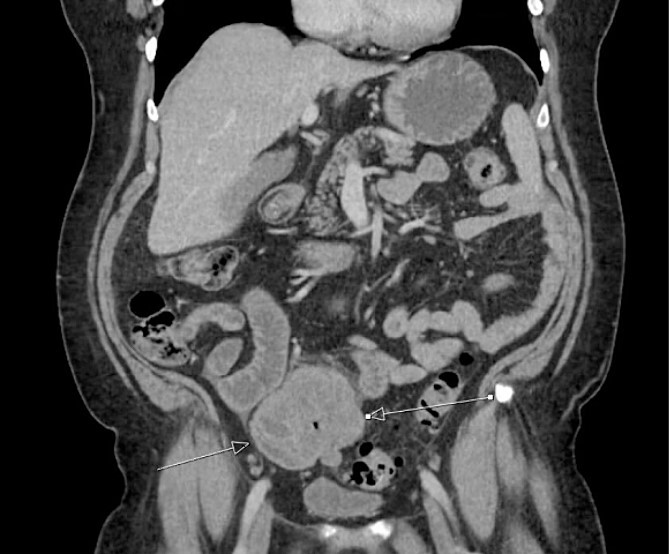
Initial CT imaging, coronal cut, showing necrotic mass causing small bowel obstruction.
